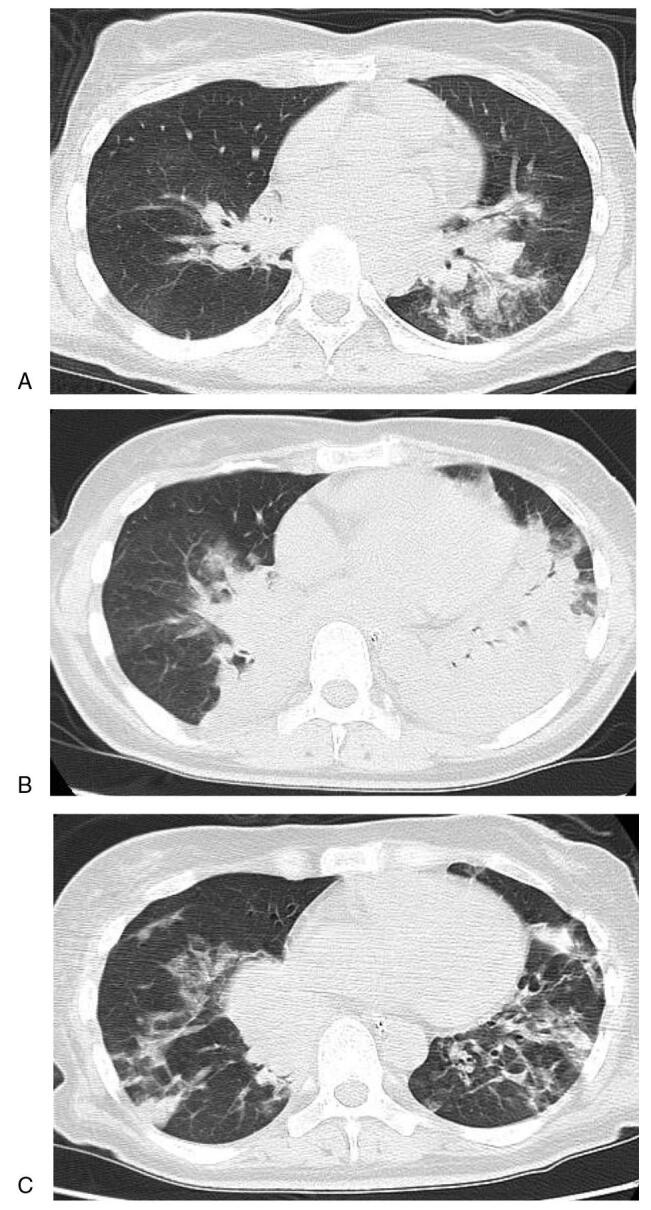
Chest computed tomography (CT) on admission (A), 2days after admission (B), 3weeks after admission (C). Chest CT on admission and at the onset of DKA showed pneumonia in the left lower lung. Chest CT taken at the onset of ARDS 2 days after admission showed bilateral dense-dependent consolidation mainly in the left lung. Chest CT taken 3weeks after admission showed consolidation shadow after pneumonia.
